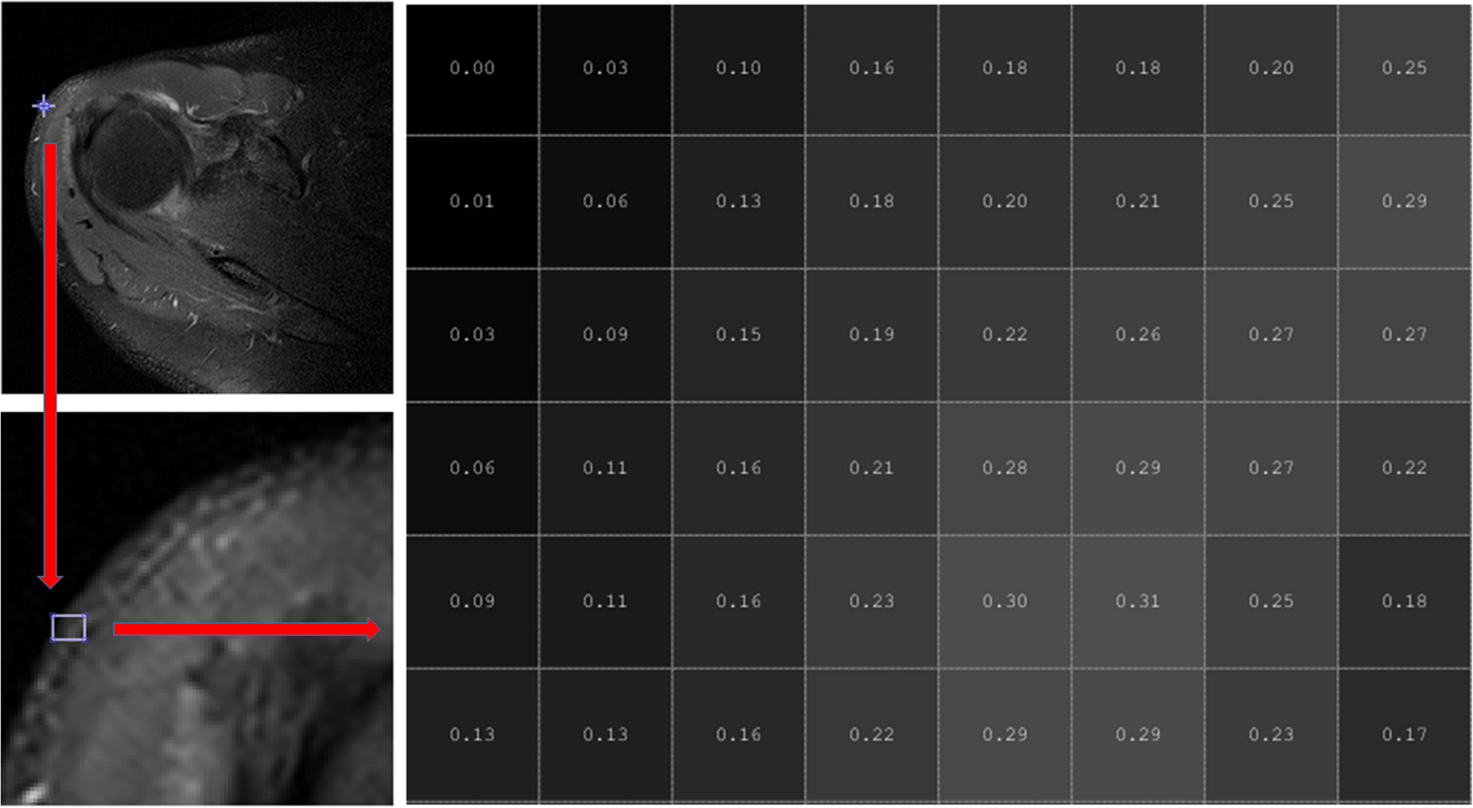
Pixel values of a region of one slice of a rotator cuff MRI
